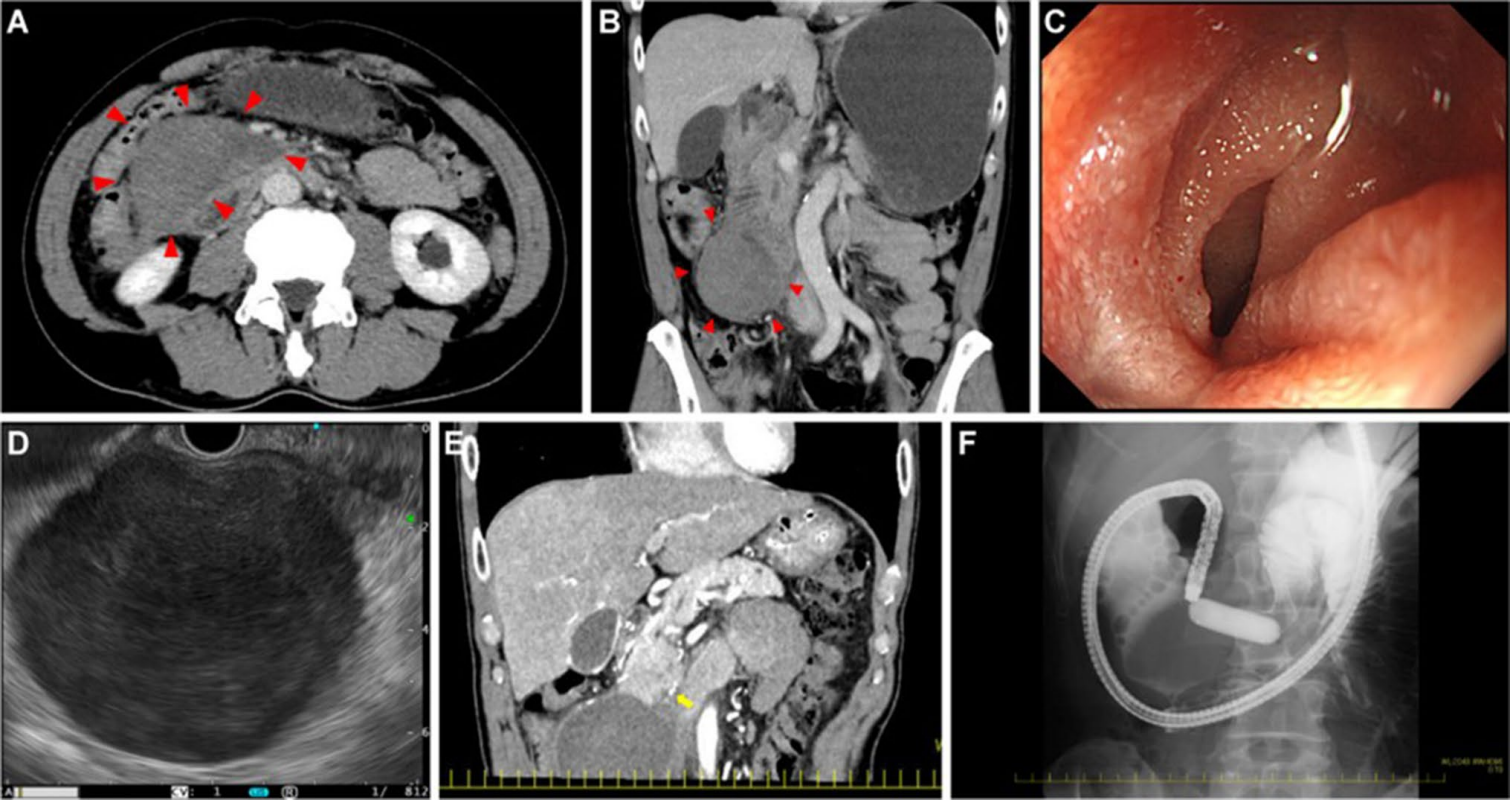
A 62-year-old male presenting with epigastric pain and vomiting. a Abdominal contrast-enhanced CT on day 1 revealed a low-density, 6-cm-sized mass (arrowheads) at the retroperitoneal space around the 2nd to 3rd part of the duodenum. b In coronal reformatted images, the inferior duodenal angle to the 3rd part of the duodenum was compressed due to the mass (arrowheads) and caused the distention of the stomach and 1st to 2nd part of the duodenum. c EGD on day 2 revealed stenosis of the 3rd part of the duodenum. Although the upper endoscope did not pass through the stenosis due to its bending in the stomach, a short-type, balloon-assisted endoscope could pass through there. No neoplastic lesion was identified on the mucosal surface. d EUS was performed after the EGD. A 7-cm-sized mass with a clear margin was observed just outside of the 2nd part of the duodenum The mass showed low echogenicity with a partially anechoic area and a debris-like area of slightly high echogenicity. e Contrast-enhanced computed tomography on day 14 revealed no change in the size of the hematoma. A pseudoaneurysm of the posterior inferior pancreaticoduodenal artery (yellow arrow) was suspected in the left edge of the hematoma. This image was previously used in a Japanese case report ([6]). f EGD on day 23 still revealed duodenal stenosis. The patient underwent endoscopic balloon dilation on the stenosis
